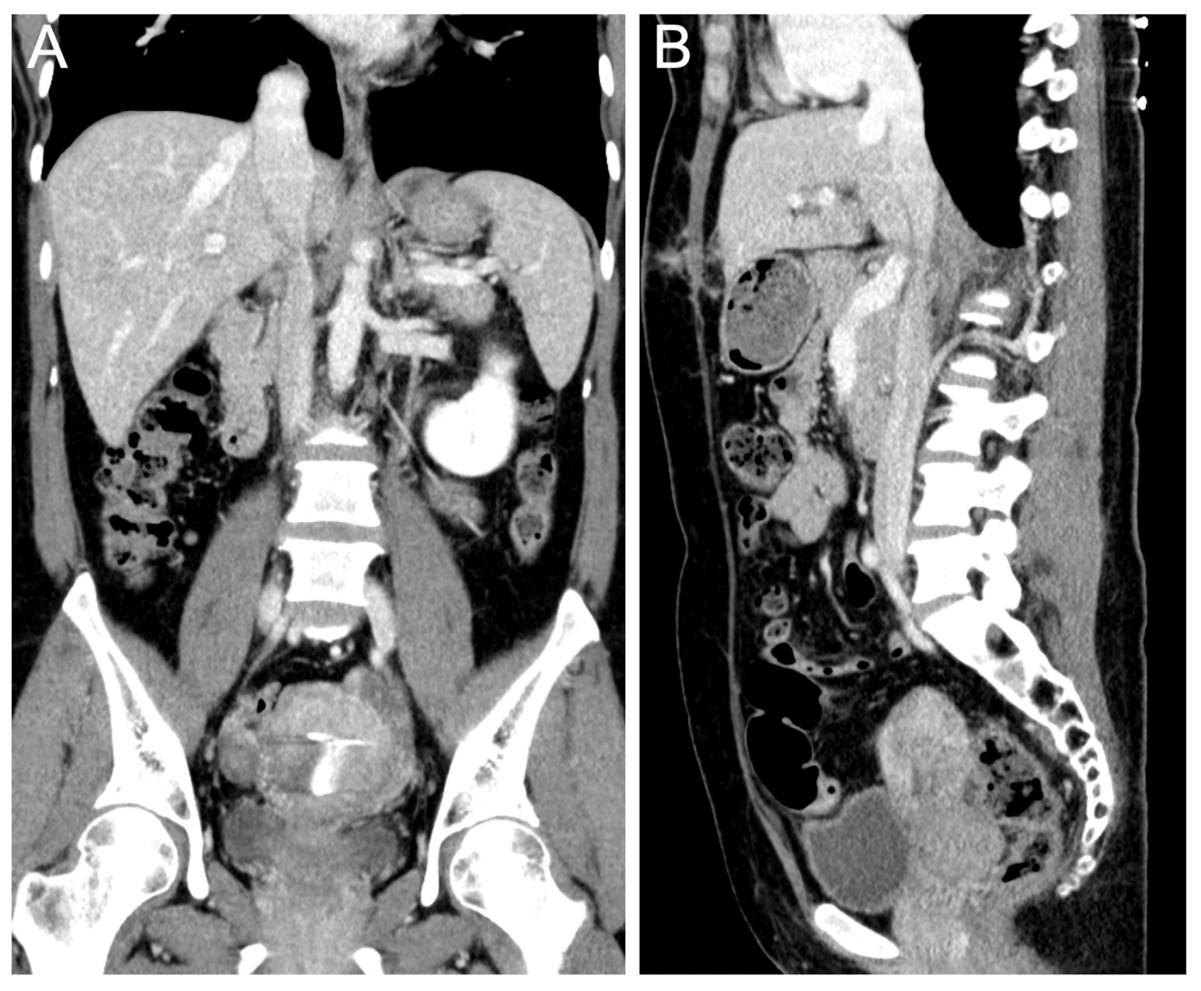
The six-month follow-up CT scan shows no recurrent tumors. (A) The coronal view of the follow-up CT scan shows no recurrent tumors. (B) The sagittal view of the follow-up CT shows no recurrent tumors.
